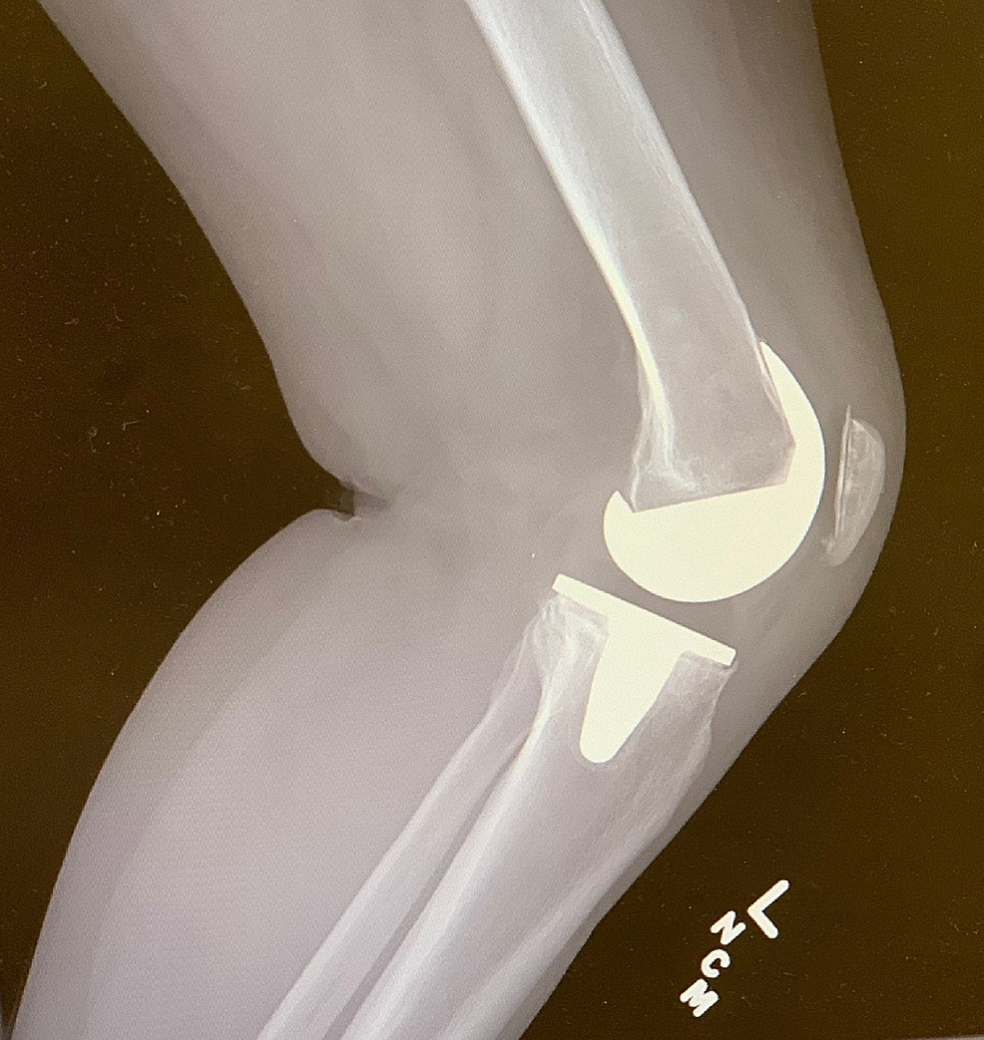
Preoperative lateral radiograph of the left knee demonstrating no obvious signs of aseptic loosening of the components.
